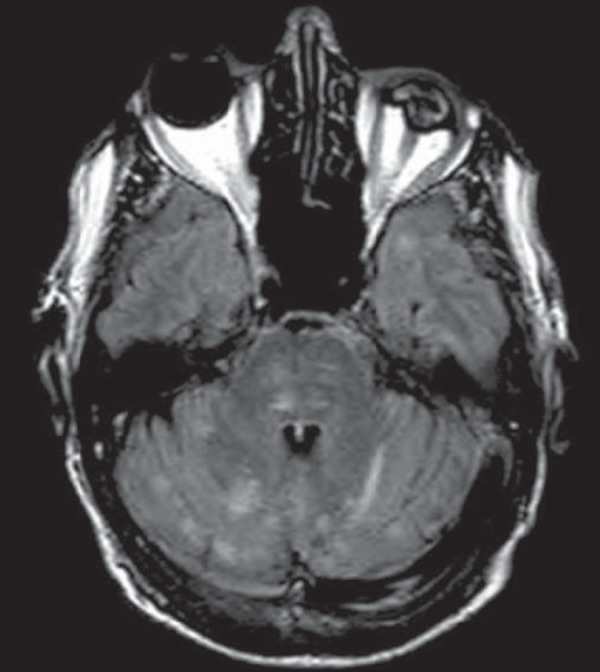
Listeria*. Axial FLAIR MRI sequence showing
